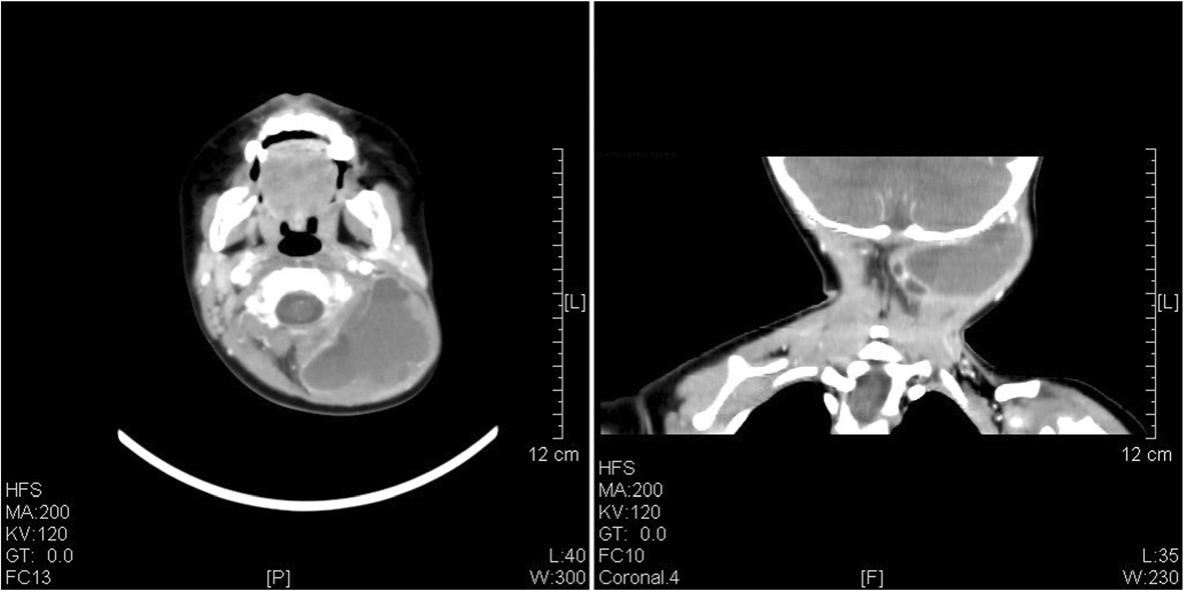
A contrast CT scan revealed a low-density lesion without enhancement in the left occipitalia in case 1.
